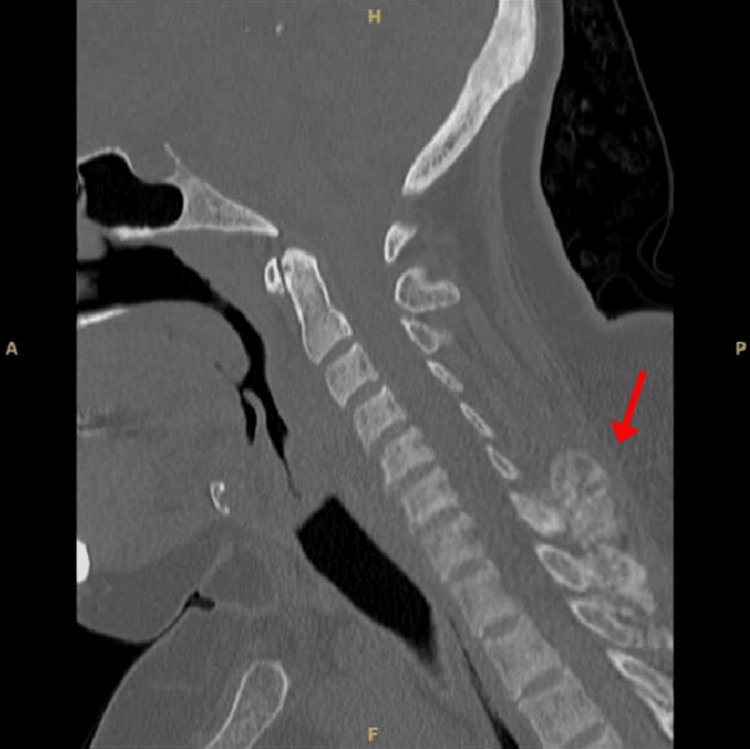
CT sagittal view of the spinous process involvement.
